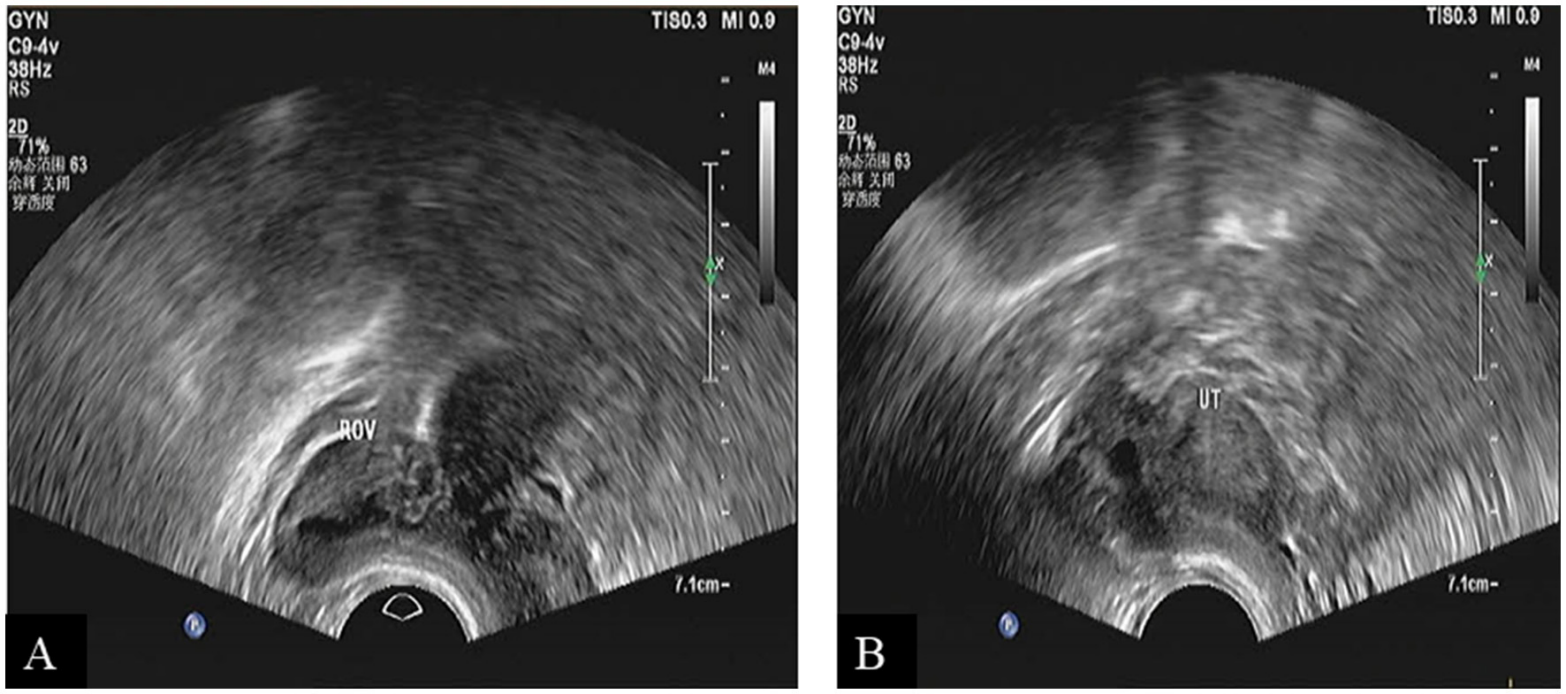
Ultrasound scan showed a unicornuate uterus during follow-up. (A) Right ovarian imaging. (B) Uterine imaging.
